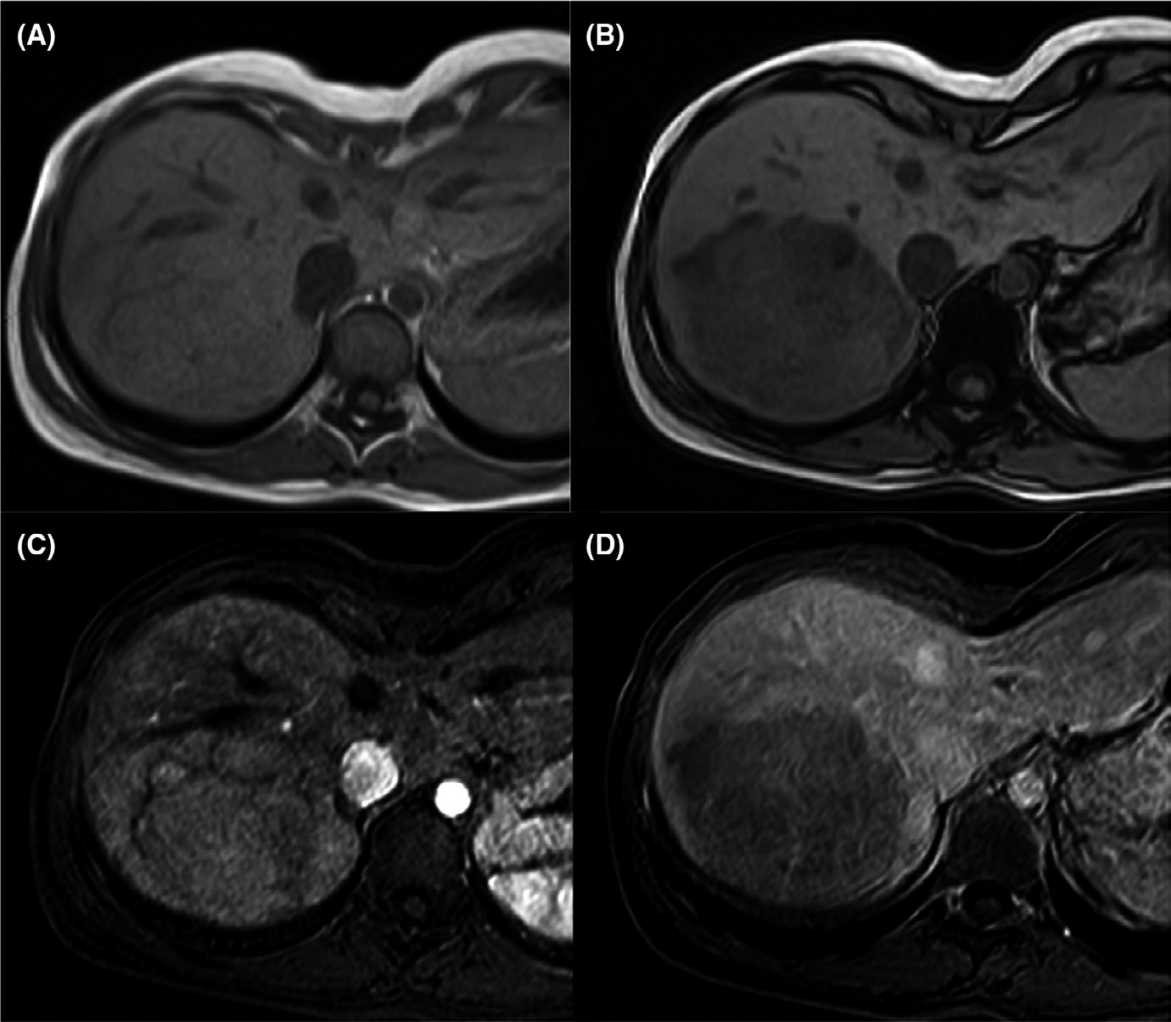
H‐HCA in a 23‐year‐old woman. MR images show a large lesion in the right liver lobe. The MR shows the presence of diffuse fat deposition within the lesion (drop of the signal on opposed‐phase T1‐weighted image B—if compared to in phase image—A). The lesion slightly enhances on hepatic arterial phase (C) and shows washout on portal venous phase (D)
